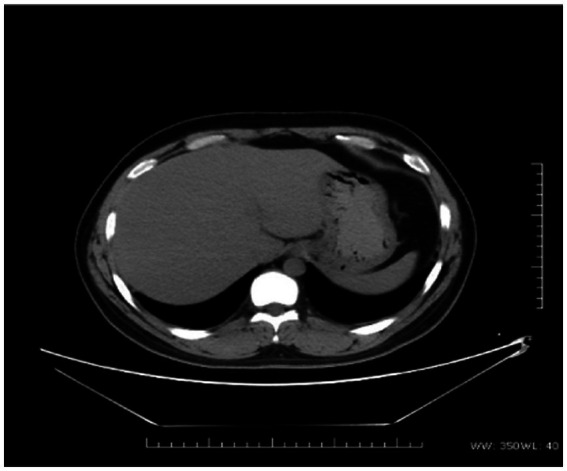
The CT scan of the proband. Lower density and normal shape of the liver (CT index is 50 U).
